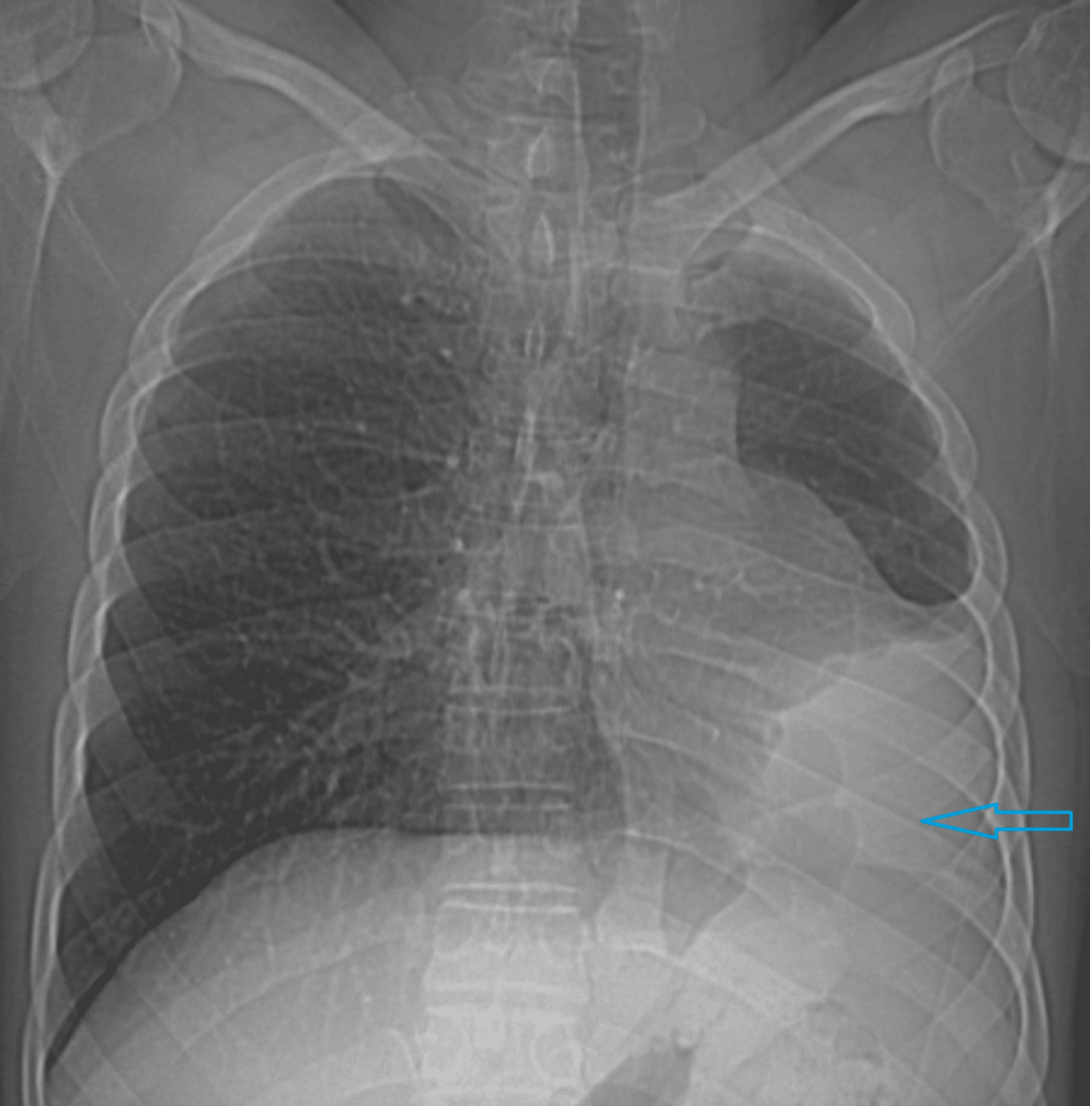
Chest radiograph in posteroanterior view shows opacity in the left lower hemithorax with ipsilateral mediastinal shift and elevated left hemidiaphragm
